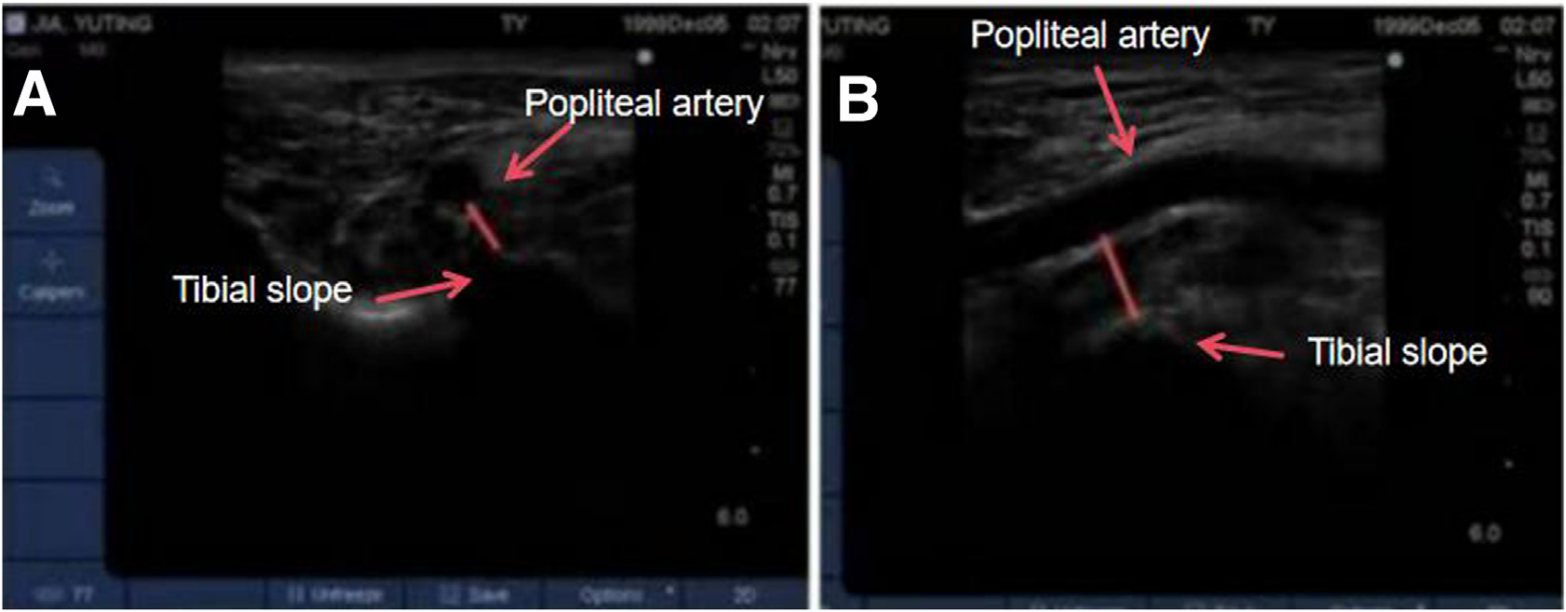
(A) Horizontal and (B) vertical modes of ultrasound positioning with the knee flexed at 90° (side, right; position “4”).
